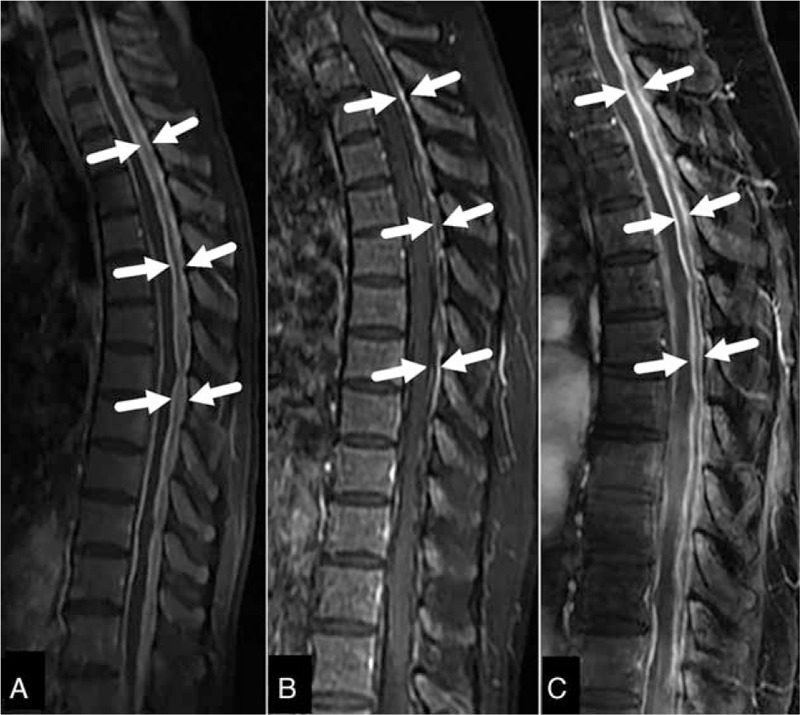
Case 1. Sagittal Gd (gadolinium)-enhanced T1-weighted magnetic resonance images (MRIs). (A) Images taken in August 2014, demonstrating diffusely thickening of dura mater at C6 and Th10 level with marked enhancement (arrow). (B) Images taken in November 2014, showing that the thickened dura mater significantly improved after immunosuppressive therapy (arrow). (C) Images taken in February 2015, demonstrating recurrence of HSP compared with previous MRI (B) (arrow).
